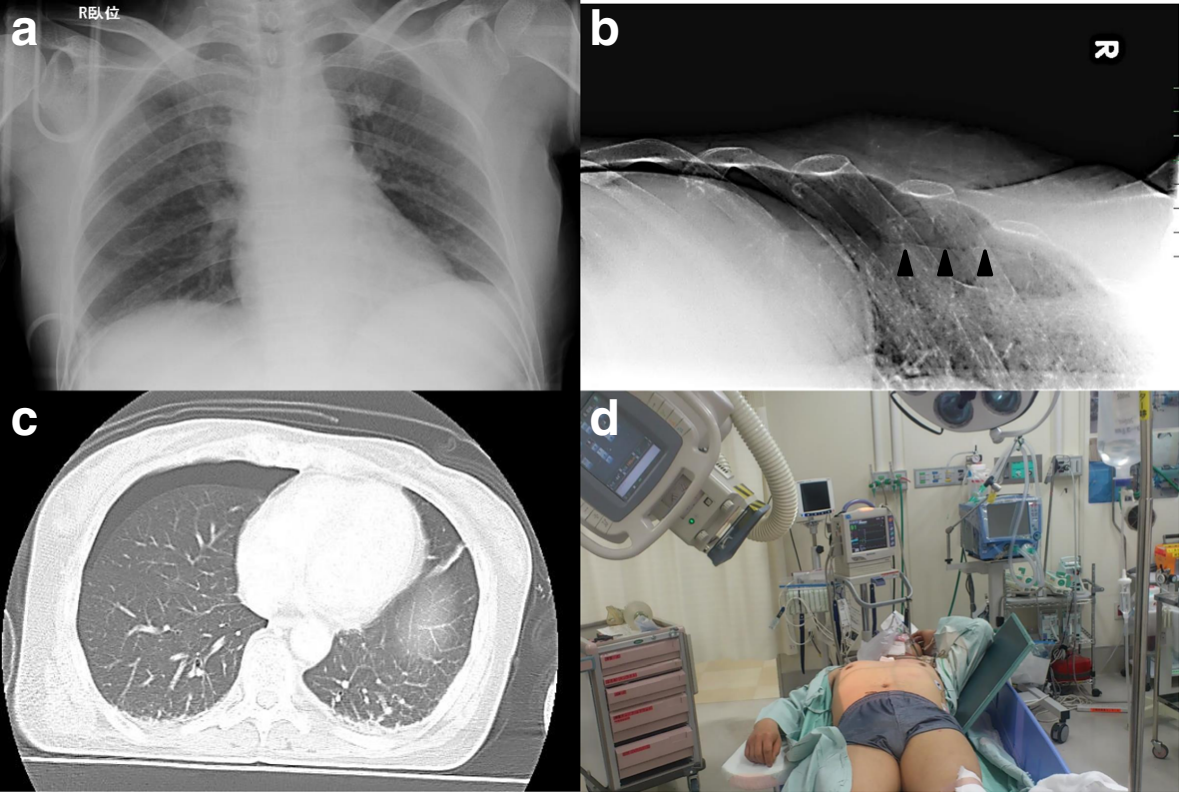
A typical case of occult pneumothorax diagnosed by OXR. This 58-year-old woman was involved in a head-on car accident and arrived with dyspnea, right chest pain. a Anteroposterior supine radiograph shows no abnormality. b OXR on the right clearly reveals a distinct visceral pleural line (arrowheads). c CT scan proves the existence of an occult pneumothorax on the right side. d Supine oblique chest radiographs could be easily performed in a trauma resuscitation area
